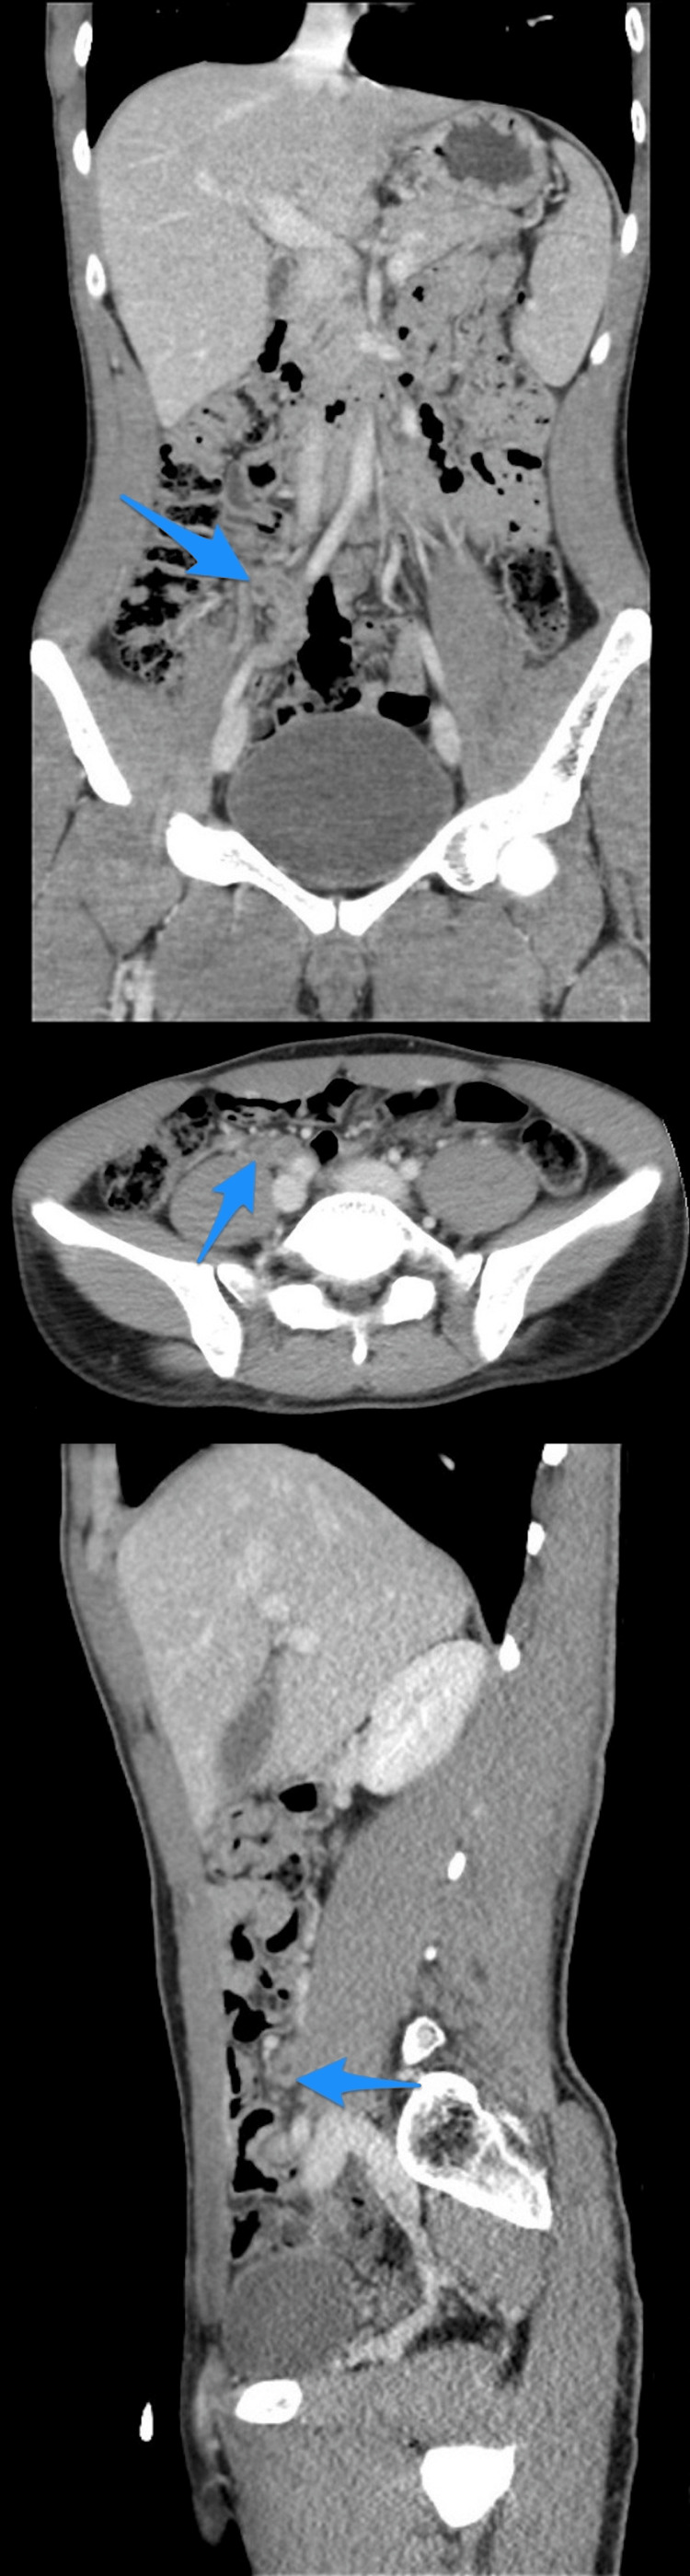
A multiview computed tomography abdominal scan (A-C) shows a small diverticulum close to the tip of an inflamed appendix (arrows).
